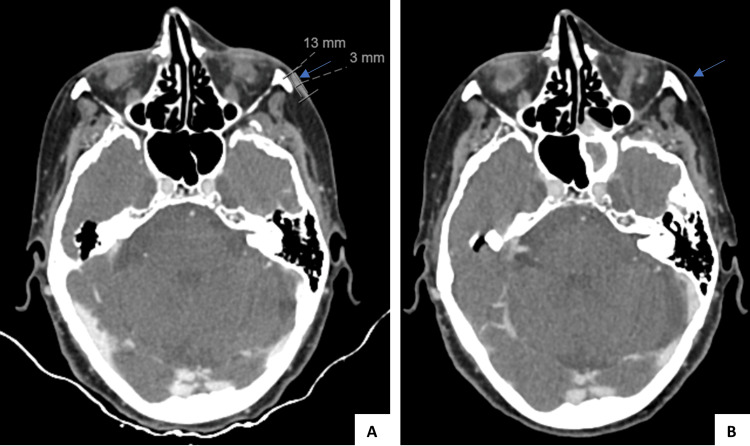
CT-scan images reporting cemiplimab response in patient four. A) Patient status at the start of cemiplimab treatment – a 13x3mm lesion on the left malar region was seen (March 2020), and B) imaging showing sustained complete response 3 years after stopping cemiplimab (February 2025).
